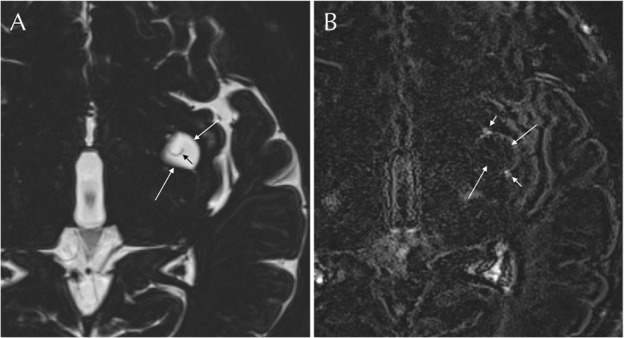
(A) Heavily T_2_-weighted magnetic resonance (MR) cisternography (TR/TE = 4400 ms/543 ms, 1 mm thick) shows the sharply delineated structure of the cerebrospinal fluid signal (long white arrows). A small vessel can be seen in the center of this lesion (short black arrow). This fluid signal structure is presumed to be a giant perivascular space (PVS). The size is 14.4 mm × 13.3 mm. (B) Heavily T_2_-weighted three dimensional-Fluid attenuated inversion recovery (3D-FLAIR) (TR/TE/TI = 9000 ms/543 ms/2250 ms, 1 mm thick) obtained four hrs after intravenous single dose administration of gadolinium based contrast agent (IV-SD-GBCA). The giant PVS does not show contrast enhancement (long white arrows), however a small nearby PVS does (short white arrows).
